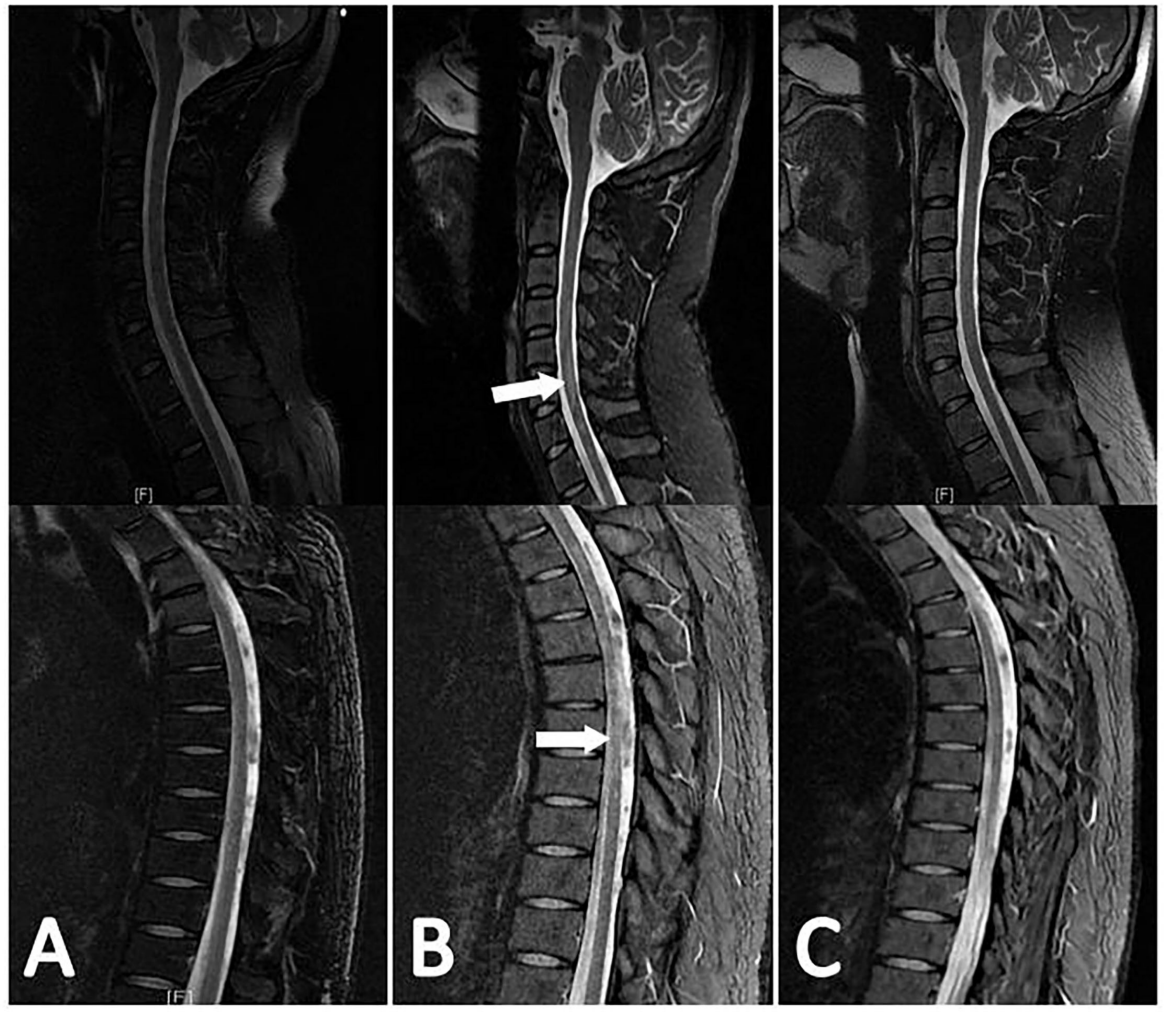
The evolution of myelitis in the same patient is shown in Figure 3. The patient's first spinal MR examination showed no abnormalities (A). 3 months later, there were multiple flaky hyperintensities in the cervical and thoracic spinal cord in the sagittal view of the T2-weighted sequence (B). 4 months later, the original lesion was absorbed (C).
